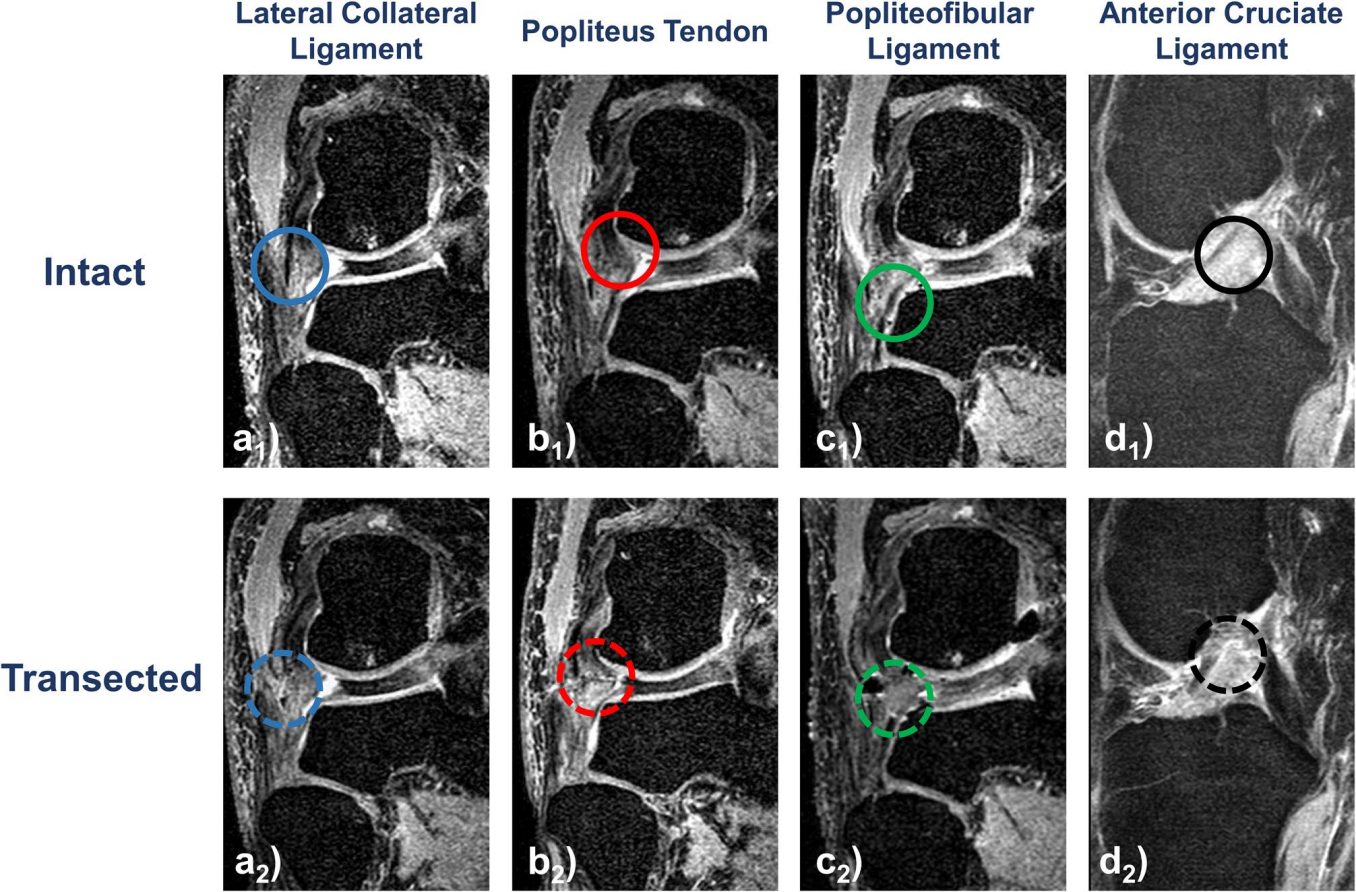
Morphologic MR images of the graded injury model. Visualized are representative coronal (a–c) and sagittal (d) images centred on the respective structure before (“intact” [a_1_–d_1_], full circles) and after sequential surgical transection (“transected” [a_2_–d_2_], dotted circles). 3D proton density-weighted fat-saturated sequence. Color-coding: lateral collateral ligament—blue, popliteus tendon—red, popliteofibular ligament—green.
